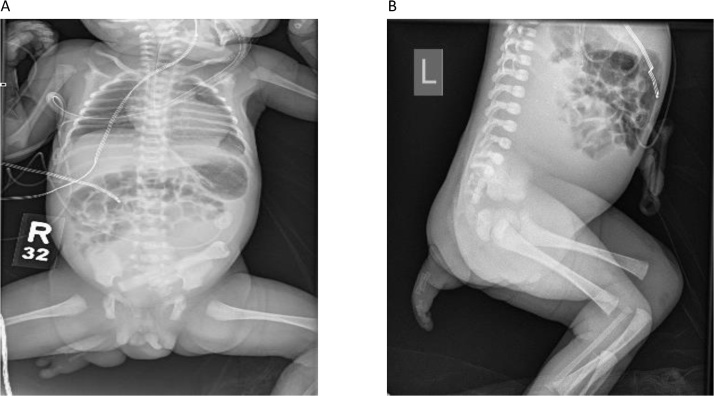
AP and lateral X-ray views of the infant chest and abdomen. A: AP X-ray view of infant’s chest and abdomen showing accessory foot from the right gluteal region having rudimentary 2 metatarsals and phalanges (2 toes), duplication of labia and a fused sacral vertebral body. B: lateral X-ray view of infant’s chest and abdomen showing accessory foot from the right gluteal region having rudimentary 2 metatarsals and phalanges (2 toes) and fused sacral vertebral body.
